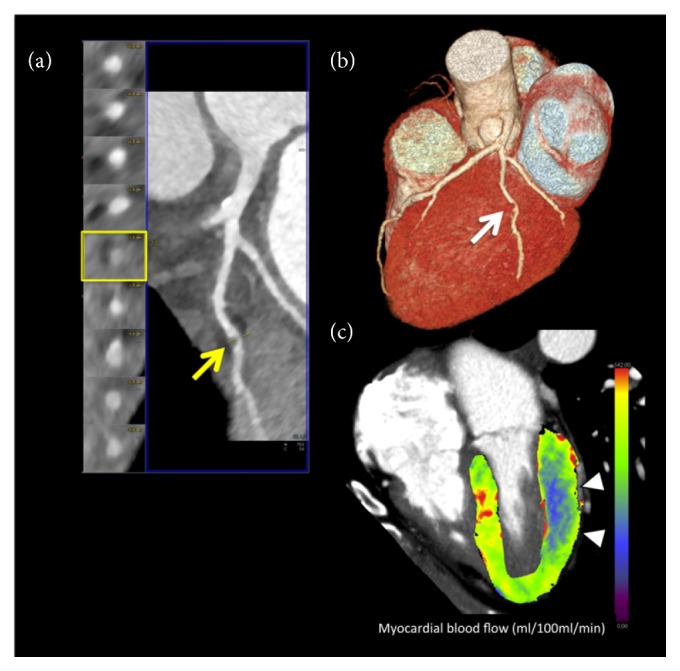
Dynamic CTP imaging. 67-year-old obese female patient with history of hyperlipidemia and smoking with suspected coronary artery disease. (a) Curved multiplanar reformation of coronary CT angiography data showed eccentric noncalcified plaque of the main obtuse marginal branch (OM) causing focal critical stenosis (>70% luminal narrowing), arrow. (b) Three-dimensional volume-rendering reconstruction confirmed the severe coronary artery stenosis of the OM (arrow). (c) Three-dimensional color-coded 4-chamber CT perfusion map image derived from the time-resolved dynamic acquisition during stress with the shuttle mode shows extensive perfusion defects in the territory of the OM (basal-middle lateral wall), color-coded in blue, arrowheads. The colors of the myocardium are coded according to the flow values with red, green, and yellow representing higher flow values than blue. The corresponding value of the hemodynamic parameters derived from the time-attenuation curves (TACs) demonstrates a significant reduction of myocardial blood flow in the territory of the OM, consistent with inducible ischemia. Absolute myocardial blood flow was 61.6 mL/100 mL/min and 118.2 mL/100 mL/min in the OM and remote myocardium (septal wall) territories, respectively.
